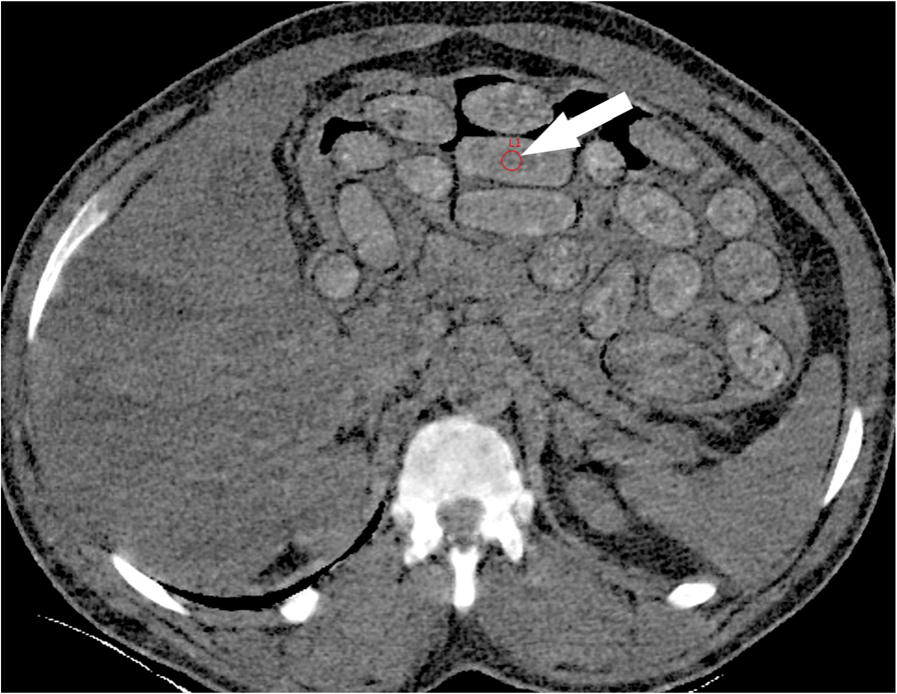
Thirty-years-old man, positive for conveying dug packets. Dual-energy CT scan shows measurement of the Z(eff) within the same drug container (*arrow*)
